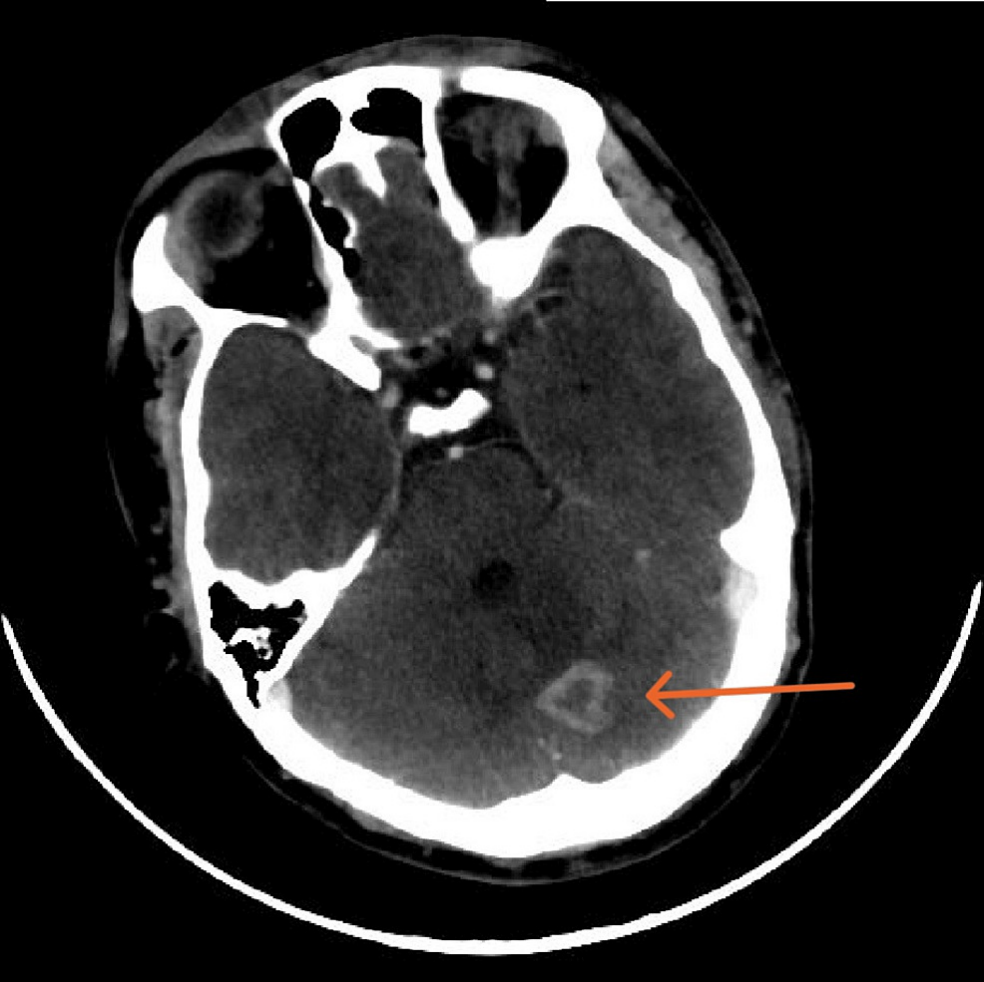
Axial cut CT of the brain demonstrating 2.2 cm × 2.3 cm ring enhancing left cerebellar lesion with surrounding edema represents tuberculoma.
